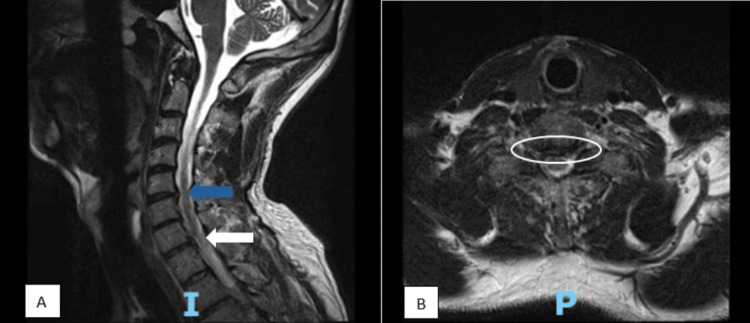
MRI of the cervical spine showing sagittal (A) and axial (B) views. (A) Sagittal view showing multiple disc herniations at C5-C6 (blue arrow) and C7-T1 (white arrow). (B) Axial view of the C5-C6 level revealing narrowing of the spinal cord (white oval).
